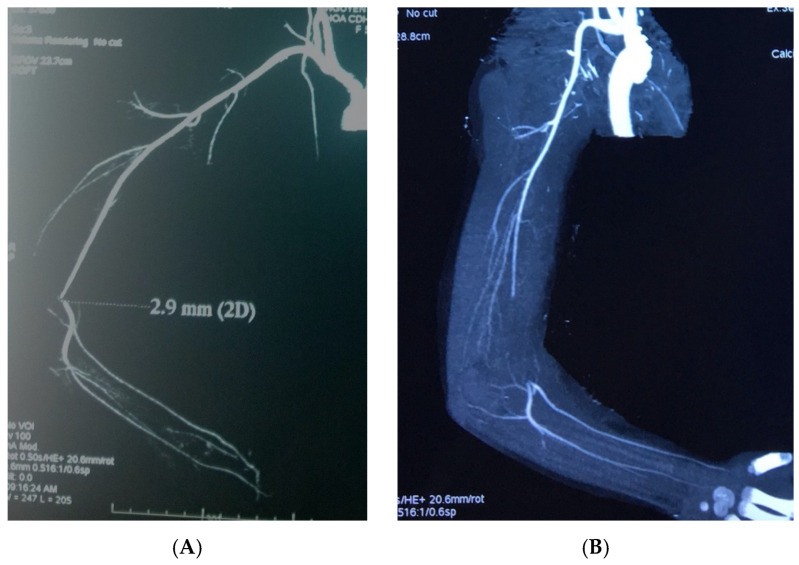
Brachial artery injuries in multislice CT-A. (A) Lesions < 5 mm. (B) Lesions ≥ 5 mm.
